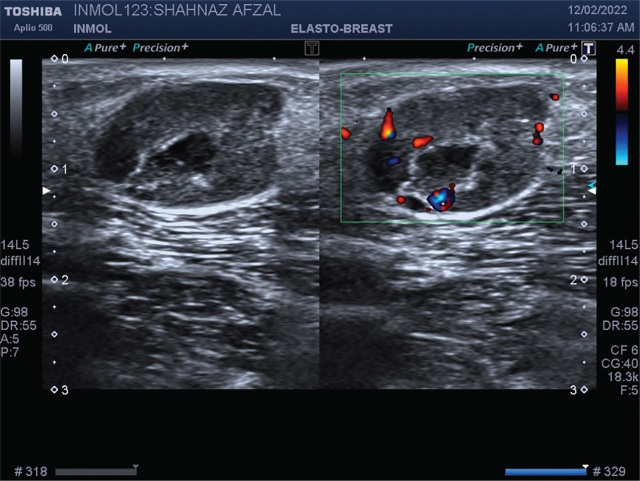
USG of cutaneous nodule revealed a 20 ×12 mm lobulated heterogeneous echogenicity lesion with internal necrotic changes in the subcutaneous plane of right axillary region, showing significant vascularity on color Doppler evaluation. USG, ultrasonography.
